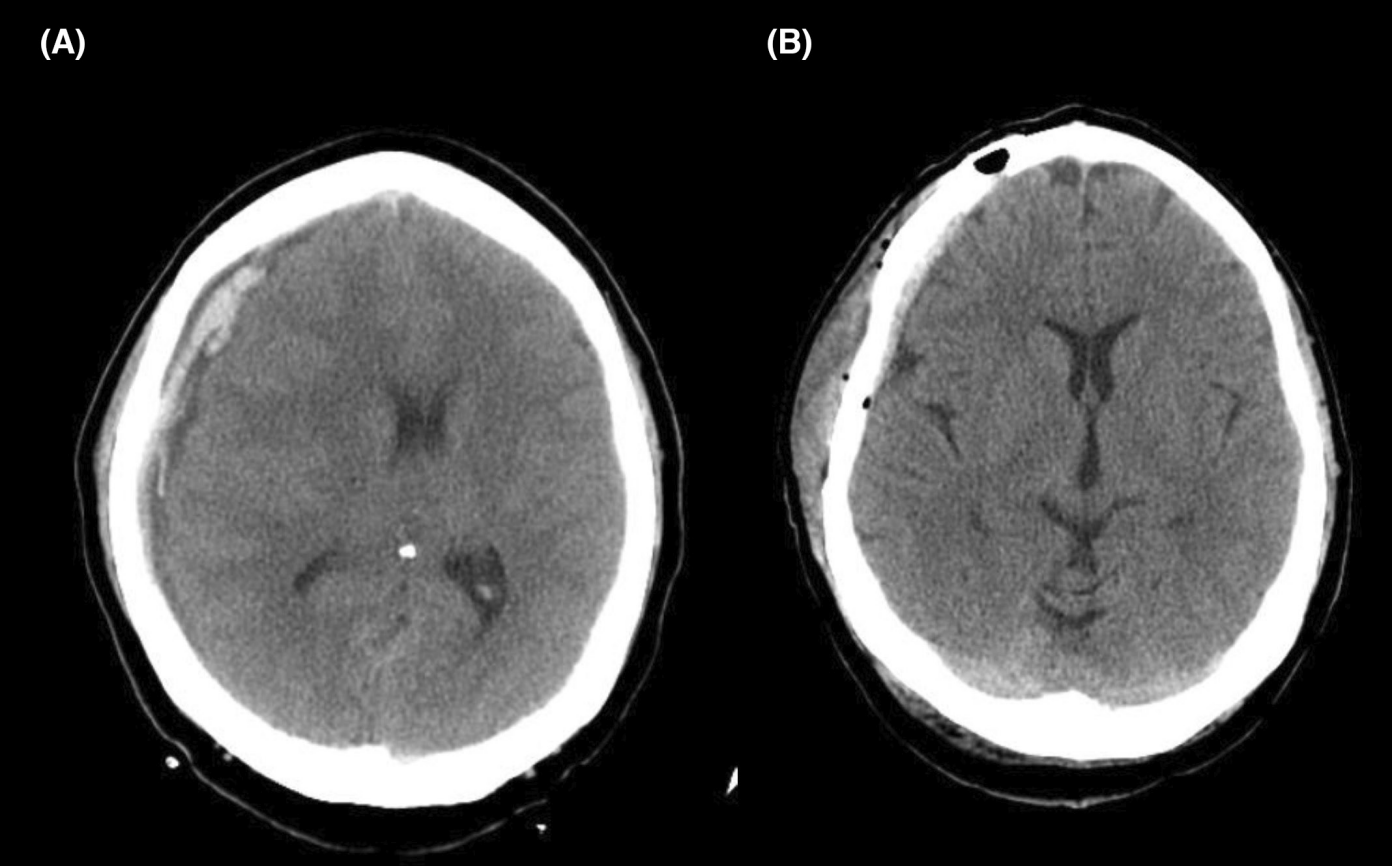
Axial brain CT‐scan of the patient after CVJ decompression revealing right frontoparietal acute sundural hematoma with 5 mm midline shift (A); postoperative axial brain CT‐scan of the patient demonstrating complete evacuation of the right subdural hematoma without midline shift (B).
